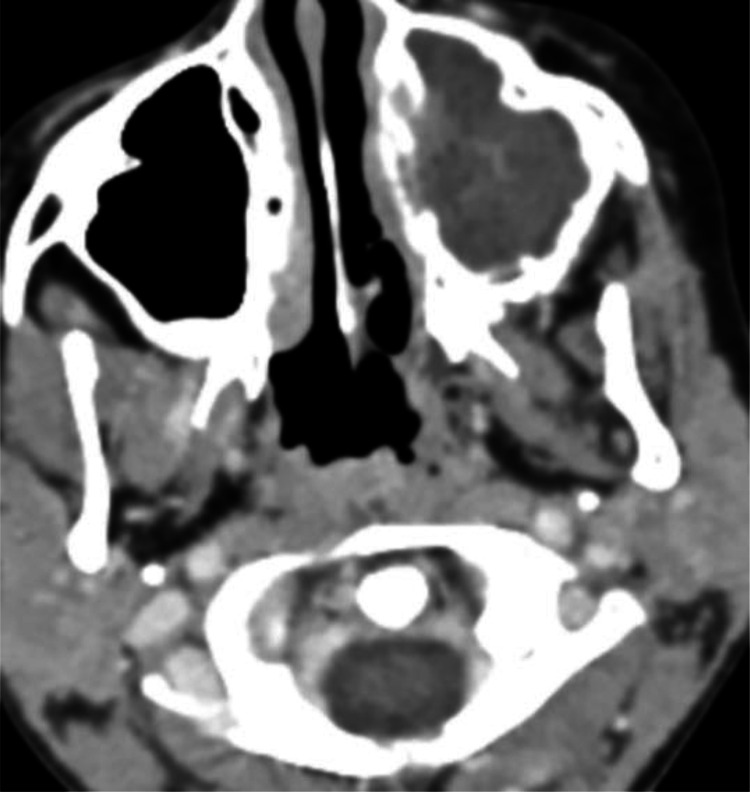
The axial section of a facial CT scan reveals a heterogeneous tissue lesion centered on the left maxillary sinus.
